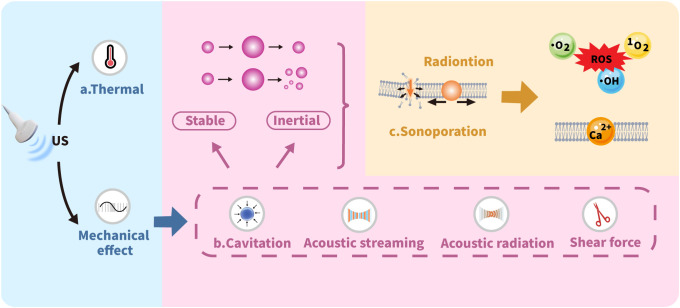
Mechanisms of ultrasound biophysical effects.
